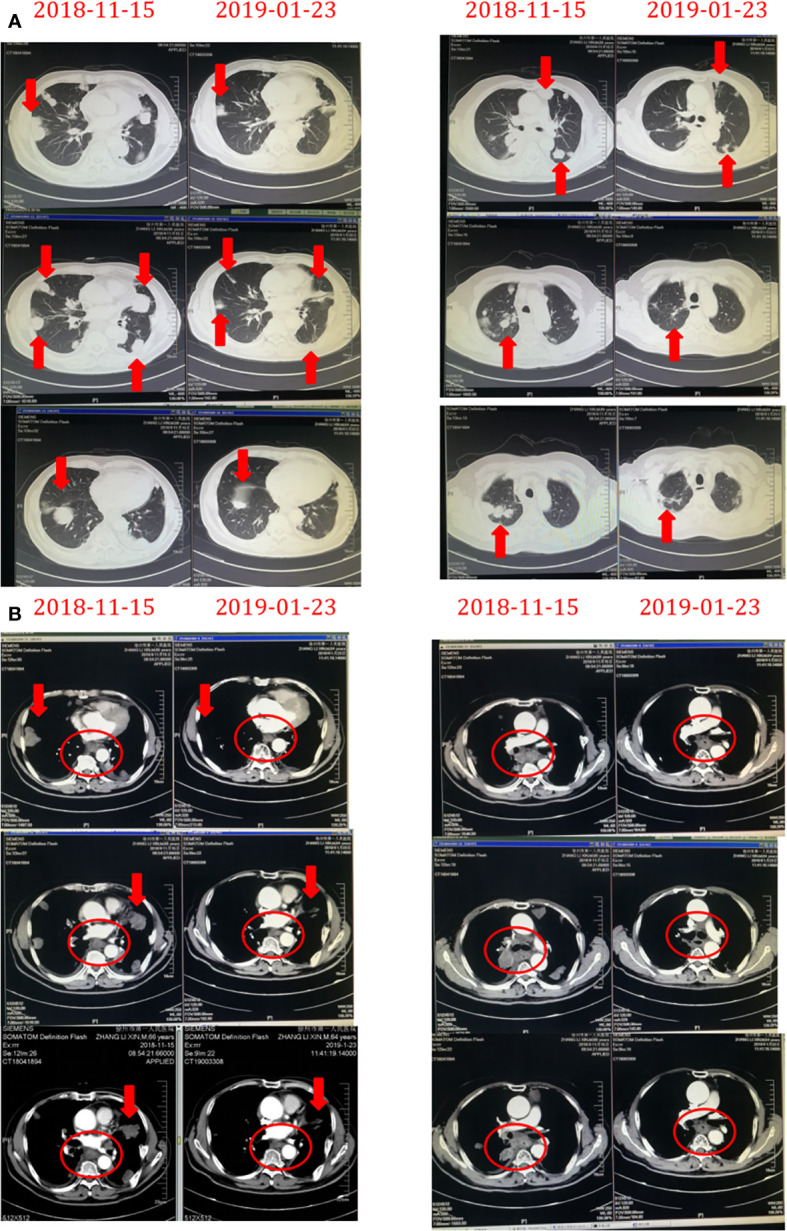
The chest masses before and after treatment with Herceptin combined with chemotherapy. The masses rapidly decreased in size on aortopulmonary window images (A) and mediastinum window images (B) derived by chest computed tomography after treatment with Herceptin combined with chemotherapy.
